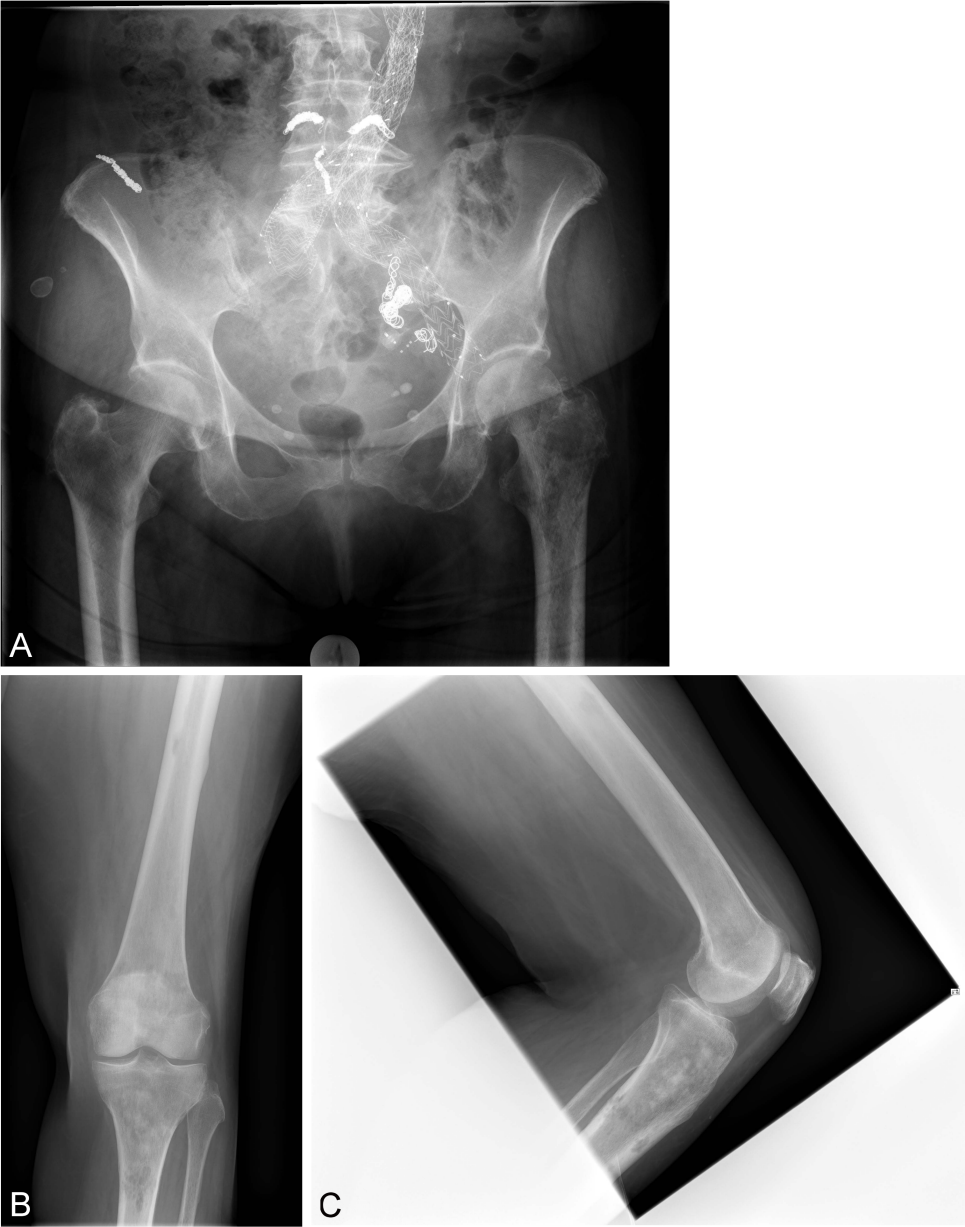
A. Preoperative X-ray of the pelvis and both hip joints: fracture of the left femoral neck with signs of infiltration of the acetabulum and of the proximal femur with metastases. B, C. Preoperative x-ray of the left knee joint, the distal femur and the proximal tibia showing signs of infiltration of the acetabulum and of the proximal femur with metastases
